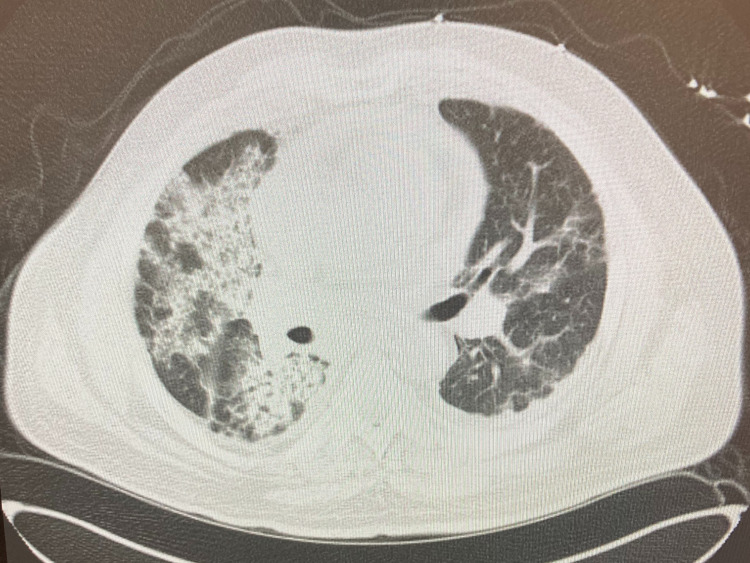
Chest computed tomography of the patient on presentation
